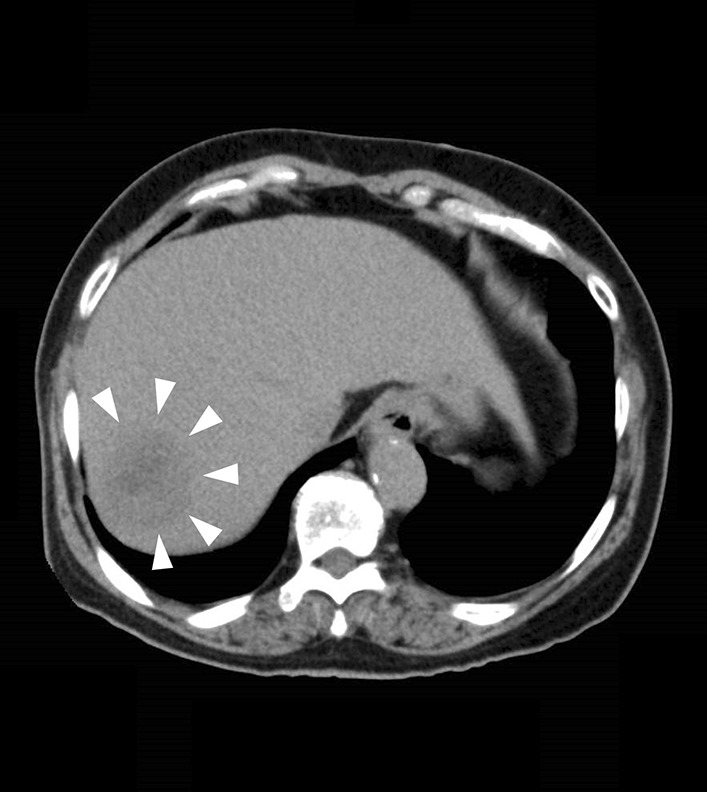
Plain computed tomography of the abdomen shows low density space-occupying lesion with largest measuring 50×37 mm (arrow head)
